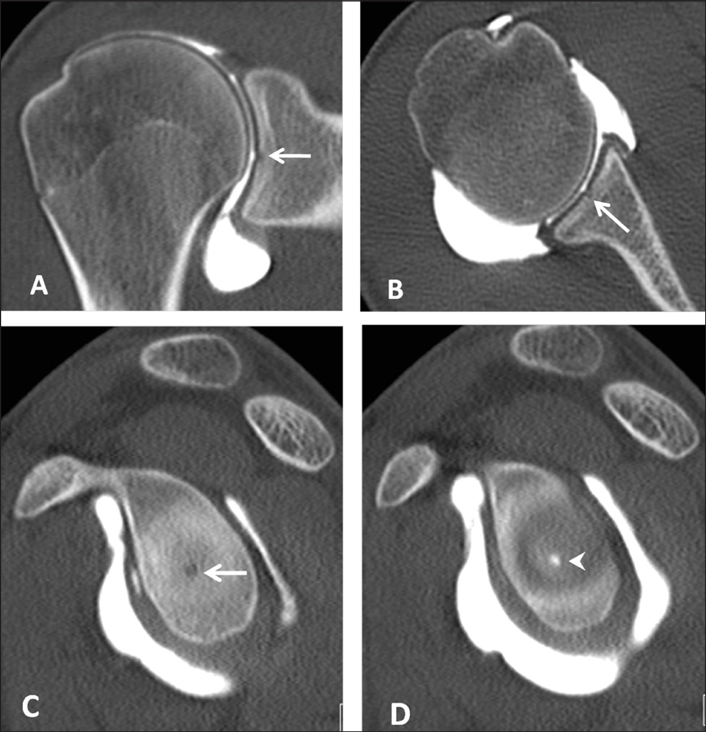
Bare area of the glenoid on CTA. (A) Coronal, (B) Axial and (C, D) Sagittal reconstructed CTA images demonstrate in the mid third of the glenoid a defect of the hyaline cartilage located centrally (arrows, A–C). On D, the defect is filled up by the injected contrast (arrowhead).
